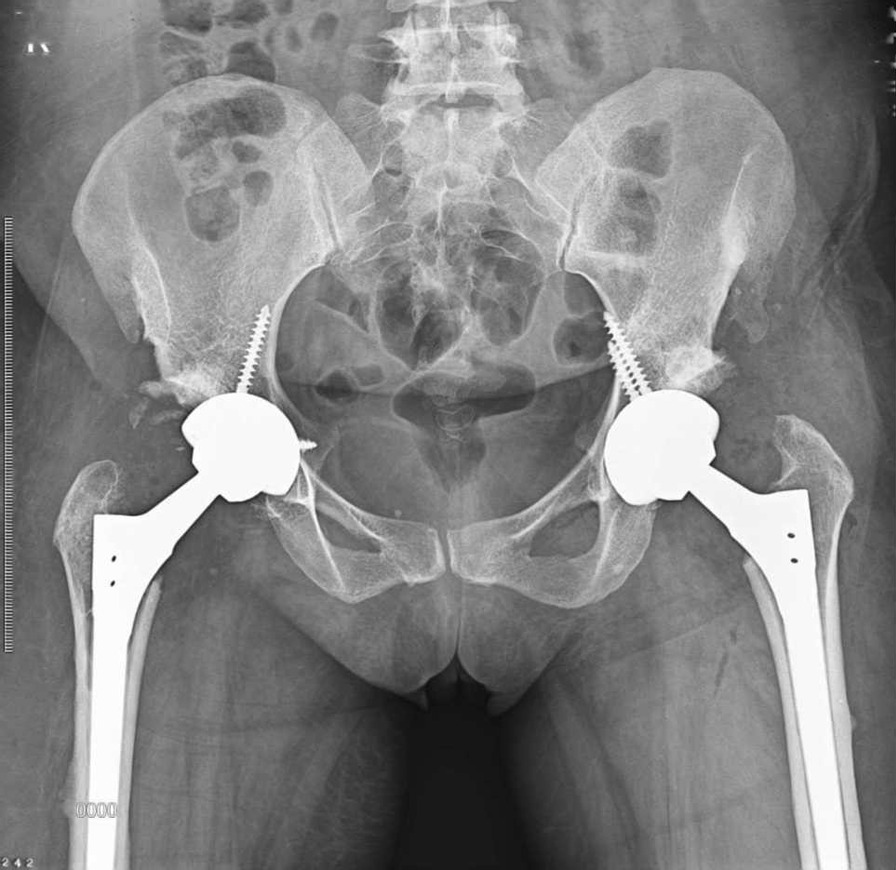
Plain hip x-ray of the patient after bilateral hip arthroplasty
